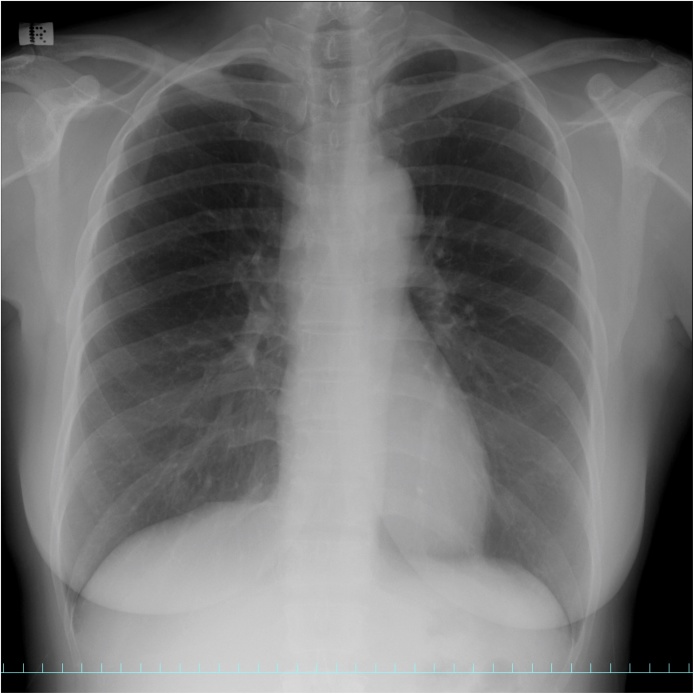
A computed tomographic scan of the chest shows an anterior mediastinal mass.
